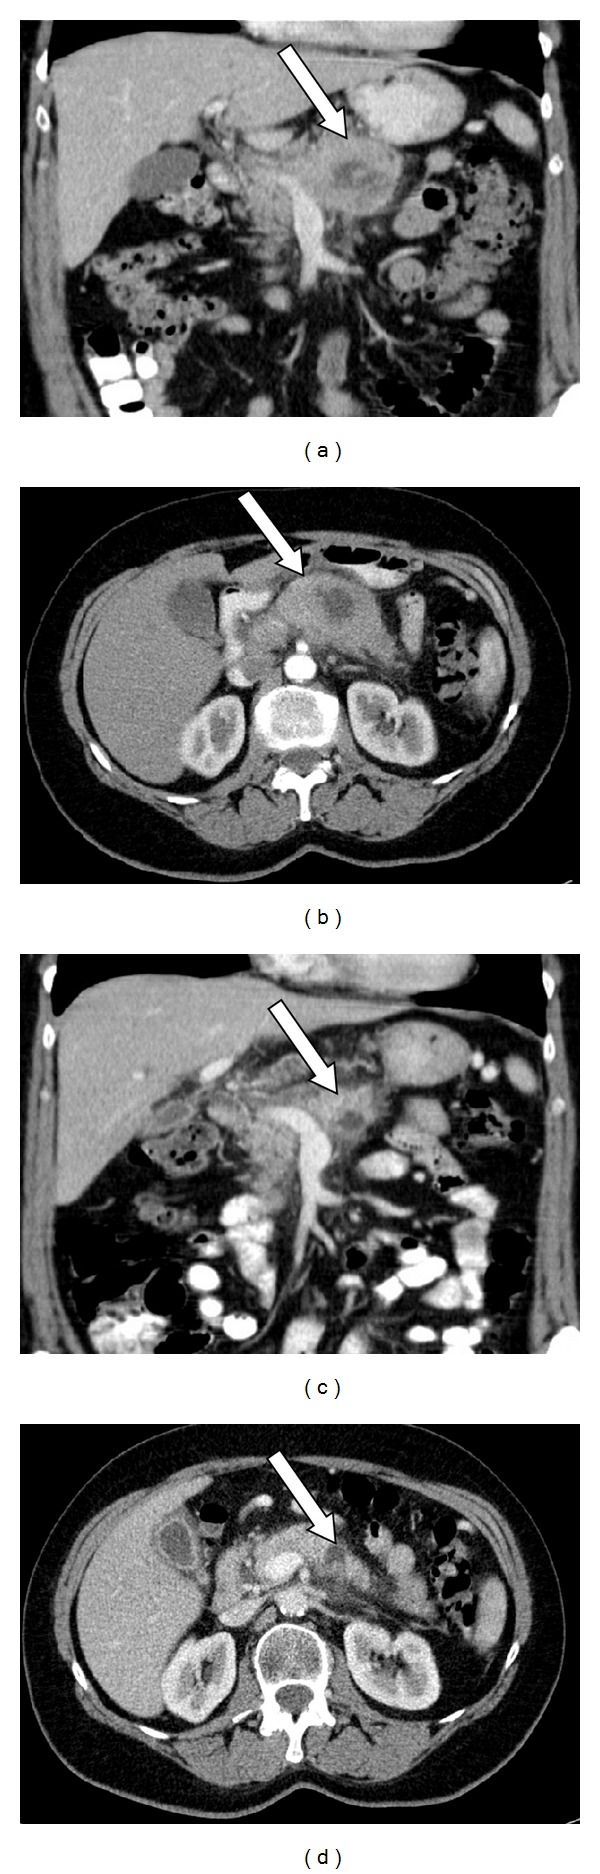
CT scans of the index patient. (a) (transversal image) and (b) (horizontal image) show the tumor's pretreatment extension with centrally cystic areas and signs of splenic vein infiltration; (c) (transversal image) and (d) (horizontal image) show tumor shrinkage after neoadjuvant radiochemotherapy; arrow is directed to tumor mass.
